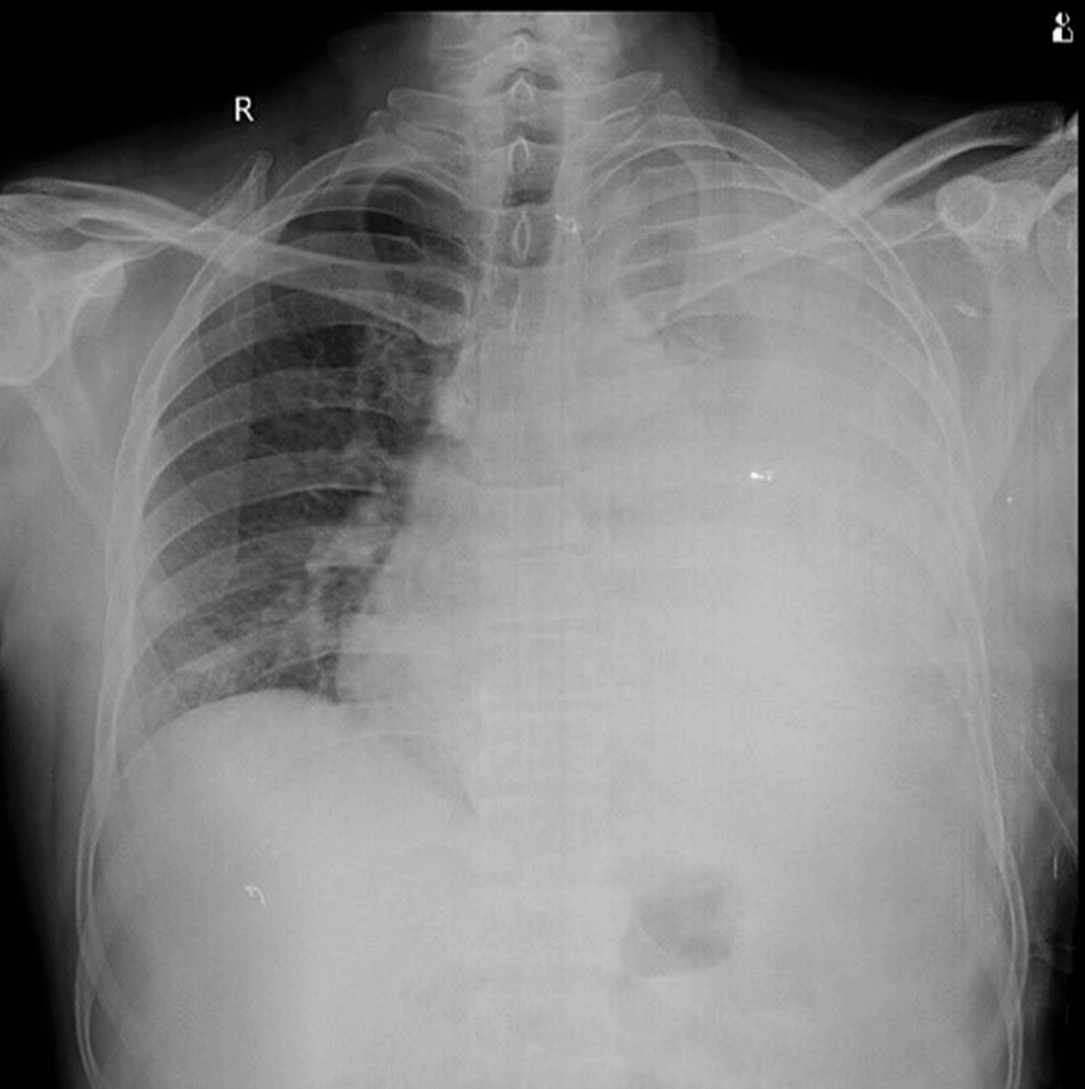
Chest X-ray post-intercostal tube insertion
